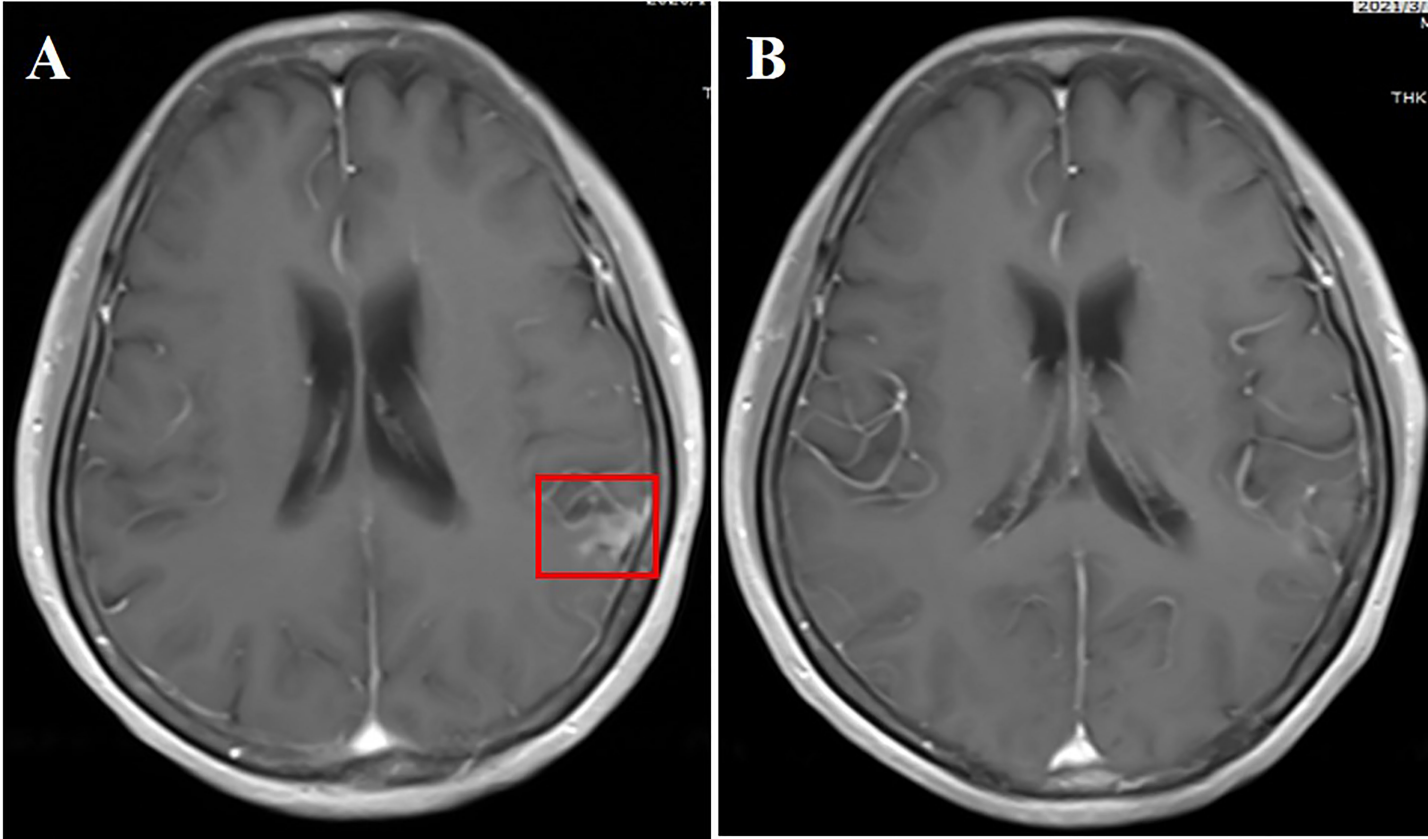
(A) Cranial enhanced MRI showing patchy high signals in the left temporal lobe (red box); (B) high signals of leptomeninges disappeared 2 months after aumolertinib plus bevacizumab treatment.
